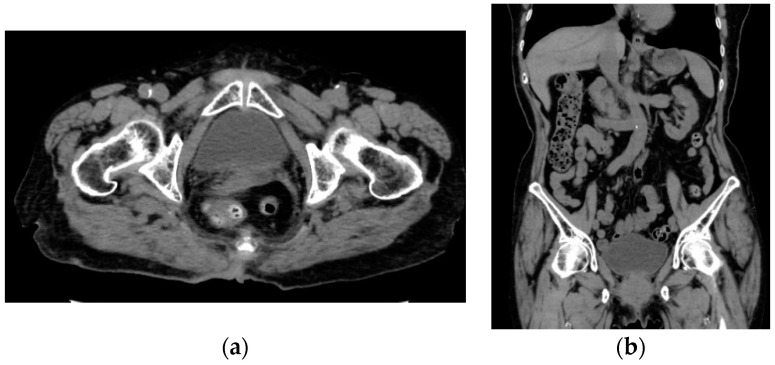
Computed tomography showing reduced recurrent lesions of (a) the vaginal stump and (b) the para-aortic lymph node.
